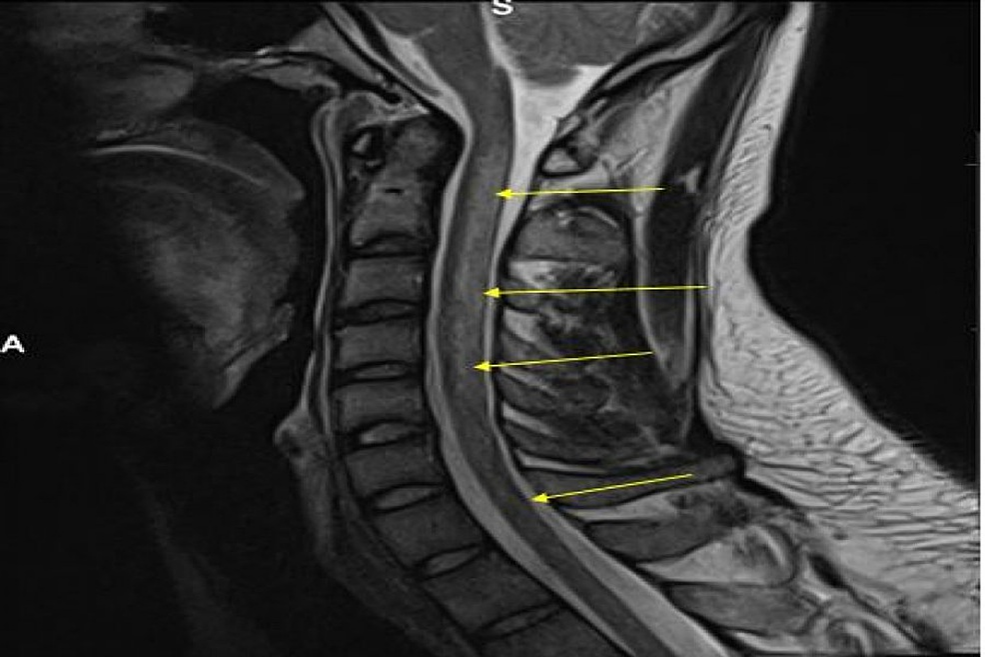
Sagittal MRI T2-weighted image showing cervical cord oedema and minimal expansion with a long segment signal abnormality discretely involving the posterior column tracts. Abnormal signal is extending up to the level of T3 (yellow arrows).
